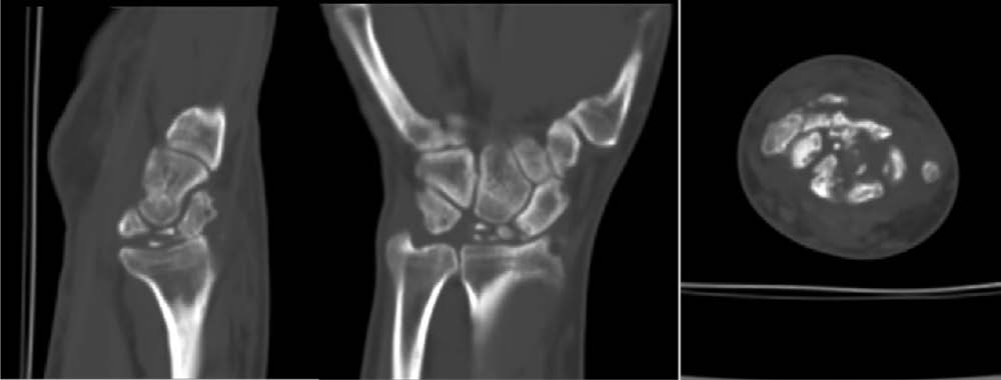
Computed tomography showing a comminuted fracture of the right lunate, a transverse fracture line in the scaphoid and styloid process of the radius.
